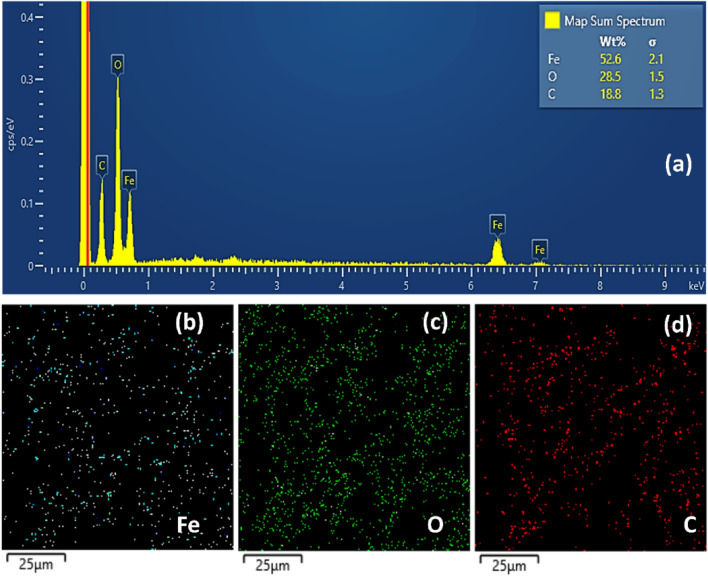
Energy dispersive X-ray pattern of GO@Fe_3_O_4_ (a), (b–d) elemental mapping of O, Fe and C elements.
